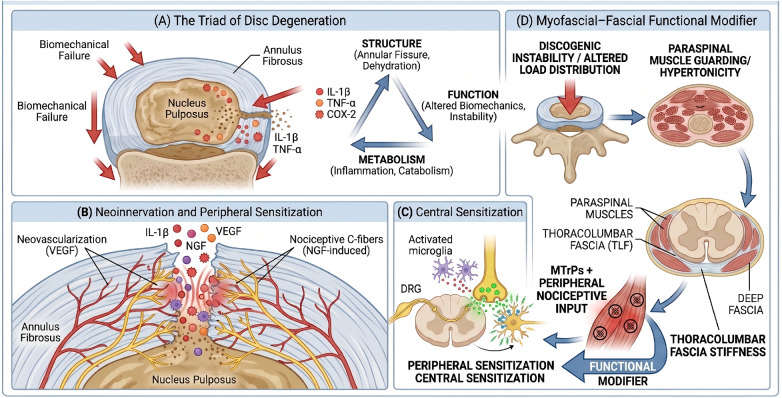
Integrated diagnostic framework for DLBP. (A) The triad of disc degeneration includes structural disruption, functional impairment/instability, and metabolic inflammation or catabolism. (B) Neoinnervation and peripheral sensitization may occur through inflammatory mediator release, neovascularization, and nociceptive C-fiber ingrowth into the annulus fibrosus. (C) Persistent peripheral nociceptive input may contribute to dorsal root ganglion activation, microglial activation, and central sensitization. (D) Myofascial-fascial functional modifiers, including paraspinal muscle guarding, myofascial trigger points, and thoracolumbar fascia stiffness, may amplify or perpetuate discogenic pain. Diagnosis requires synthesis of clinical presentation, MRI-based structural phenotyping, ultrasound-based functional assessment, and validated functional or sensitization measures. MRI evaluates Modic changes, disc signal loss, and HIZs, whereas ultrasound may dynamically assess TLF morphology, fascial gliding, shear strain, and MTrPs when functional modifiers are suspected. Patient-reported outcome measures, the Central Sensitization Inventory (CSI), and quantitative sensory testing (QST) help quantify pain severity, disability, and sensitization. Provocative discography should be reserved for selected surgical candidates when non-invasive assessments remain inconclusive.
